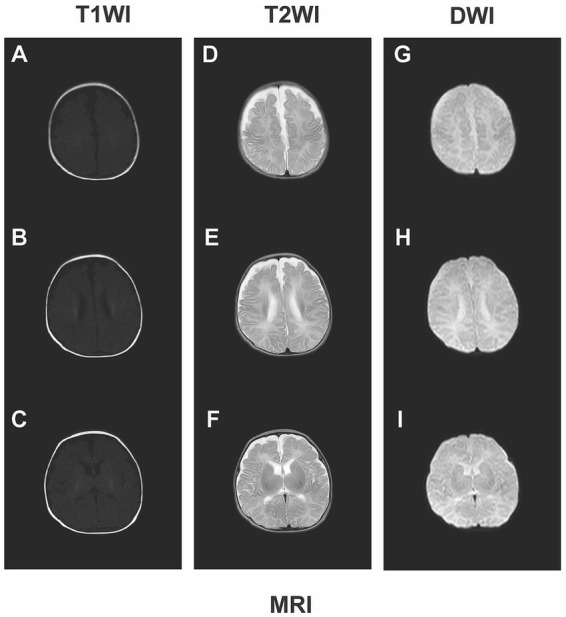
Brain magnetic resonance imaging at four months of age shows normal structure. To assess long-term prognosis, brain MRI was performed at four months of age. Axial T1-weighted (A–C), T2-weighted (D–F), and diffusion-weighted (G–I) images depict the levels of the centrum semiovale (A,D,G), periventricular region (B,E,H), and basal ganglia (C,F,I), respectively. No structural or signal abnormalities indicative of prior bilirubin-induced neurological injury were identified on any sequence.
